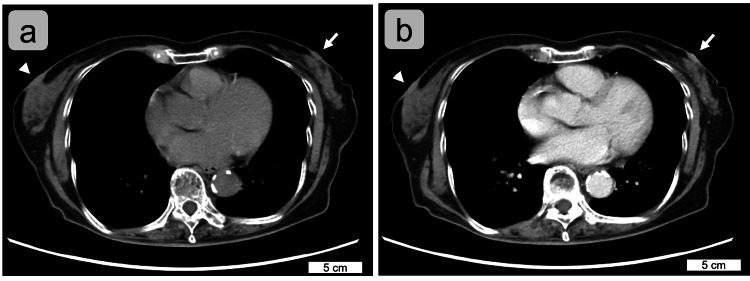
Preoperatively-taken computed tomographic images of the nipple adenoma (a) Non-enhanced and (b) contrast-enhanced images. The left nipple lesion (arrows) may be slightly enhanced compared to the right nipple (arrowheads). Scale bars are shown in the images.
